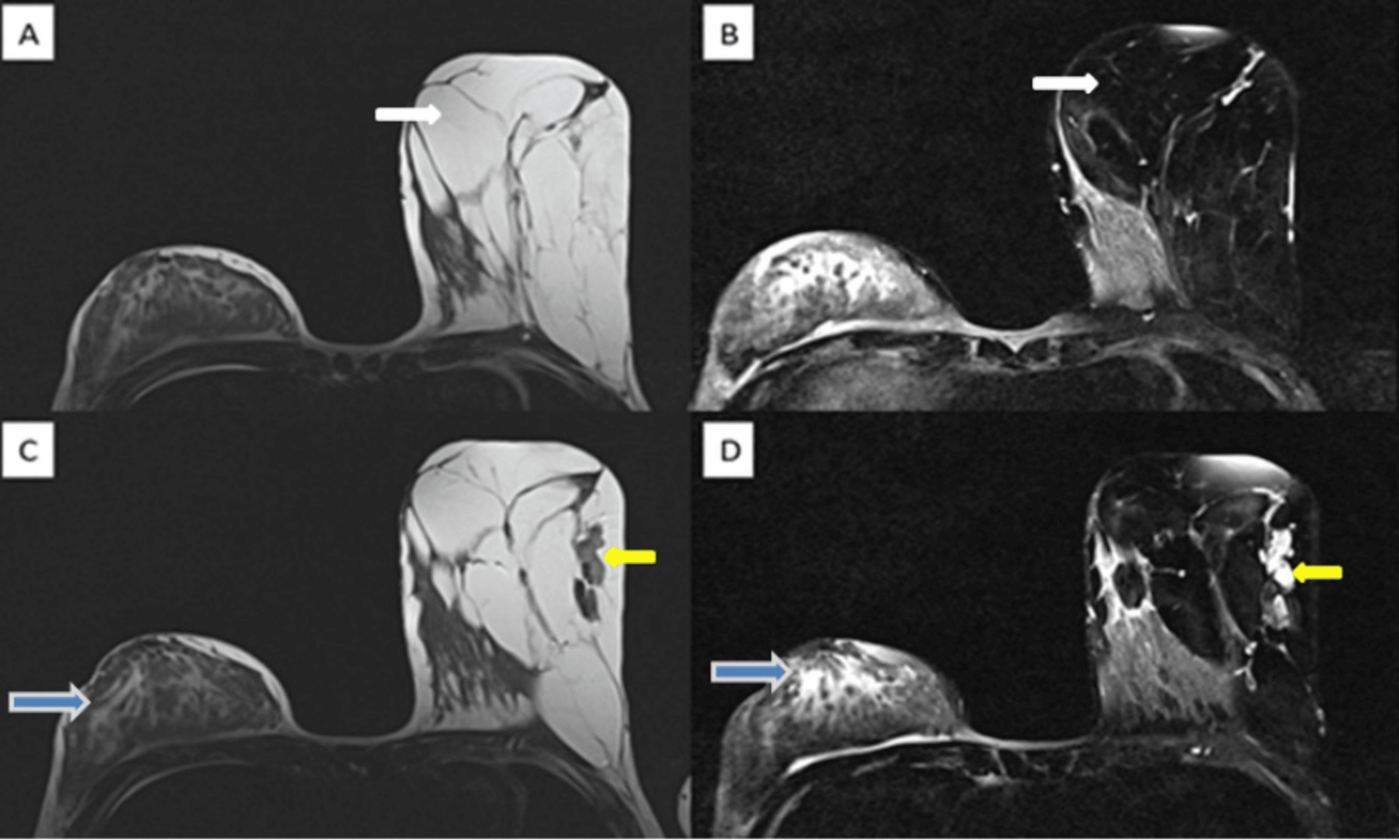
A) Axial T2WI MRI of breast showing marked enlargement of the left breast. B) Axial T2FS MRI showing the suppression of the fatty tissue, which is predominantly occupying the breast (white arrow). C) and D) Axial T2WI MRI and Axial T2FS MRI images showing an asymmetrical distribution of fibro-glandular tissue (yellow arrow). D) Normal fibro-glandular tissue seen in the contralateral breast (blue arrow).
